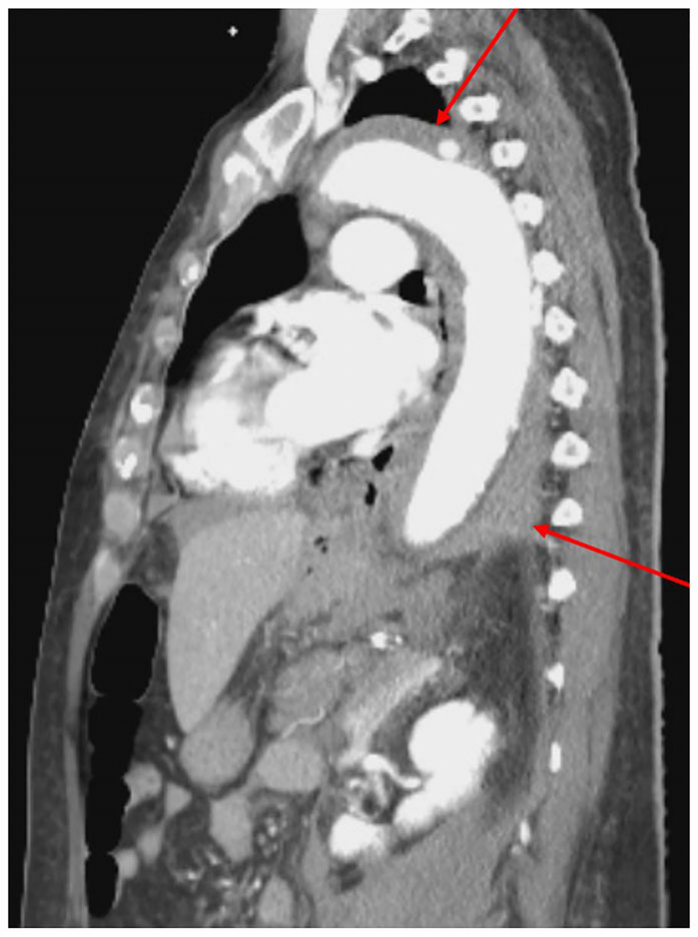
CT scan showing an intramural hematoma of the descending aorta
